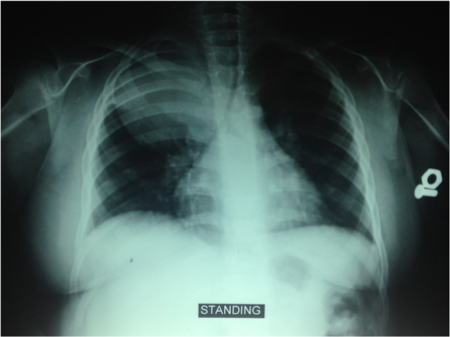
Chest X-ray reveals a big round opacity in the right lung
